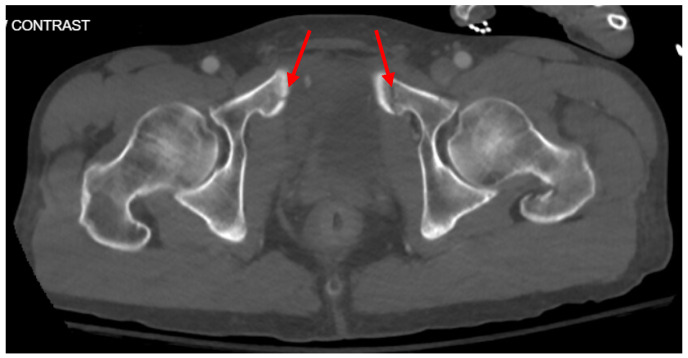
CT abdomen/pelvis images of the described fractures in case 1. Red arrows are used to indicate the location of pelvic fractures for easier identification.
